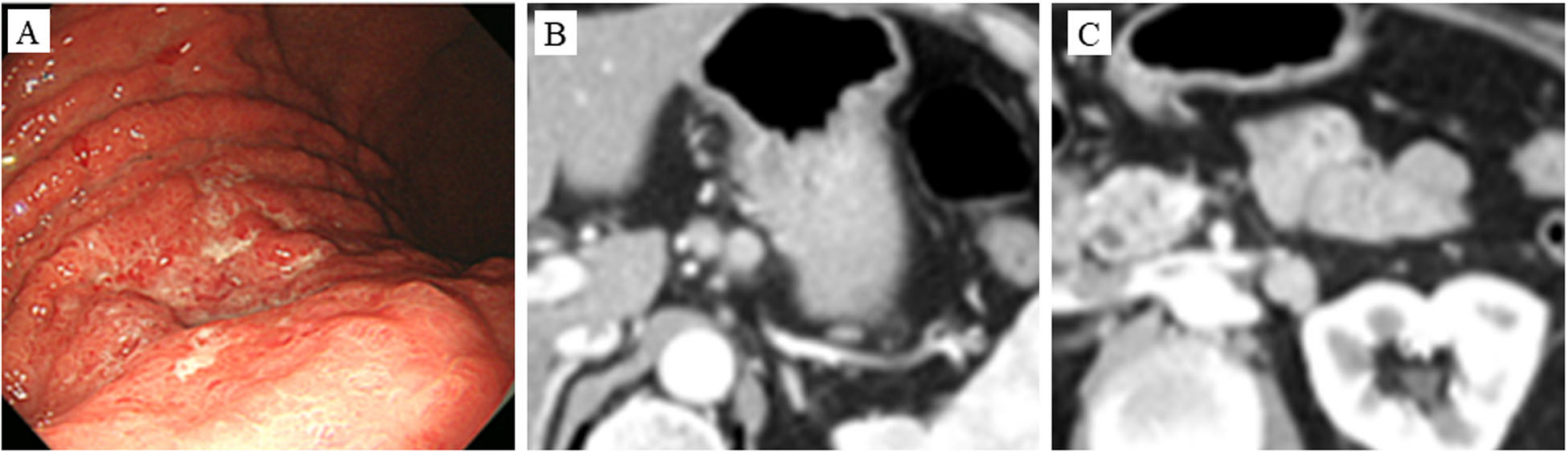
Esophagogastroduodenoscopy (EGD) findings and computed tomography (CT) findings before chemotherapy. a EGD before chemotherapy revealed Borrmann type 4 cancer at the greater curvature from the upper to middle part of the gastric corpus. Abdominal CT before chemotherapy showed swollen regional lymph nodes (b) and para-aortic lymph nodes (c)
